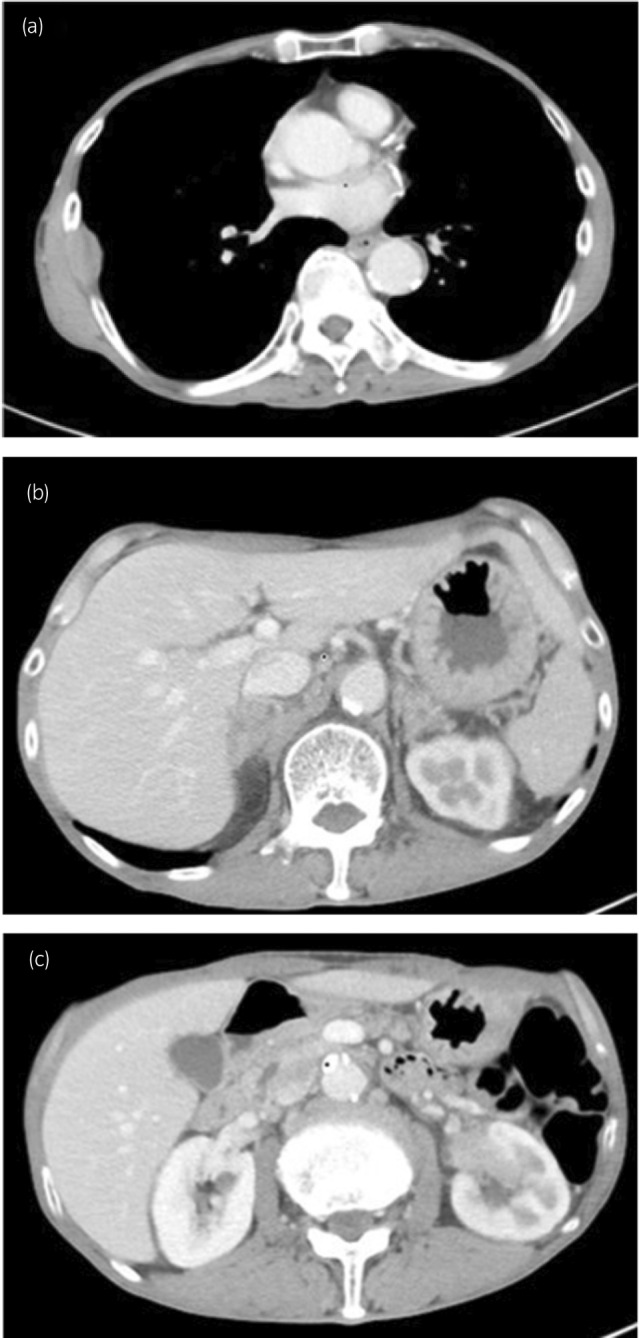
CT image on 7 months after treatment. The right thoracic wall metastasis (a) and the left kidney tumor (c) were enlarged. Both adrenal metastasis (b) remained unchanged in size. We evaluated PD using RECIST 1.1.
